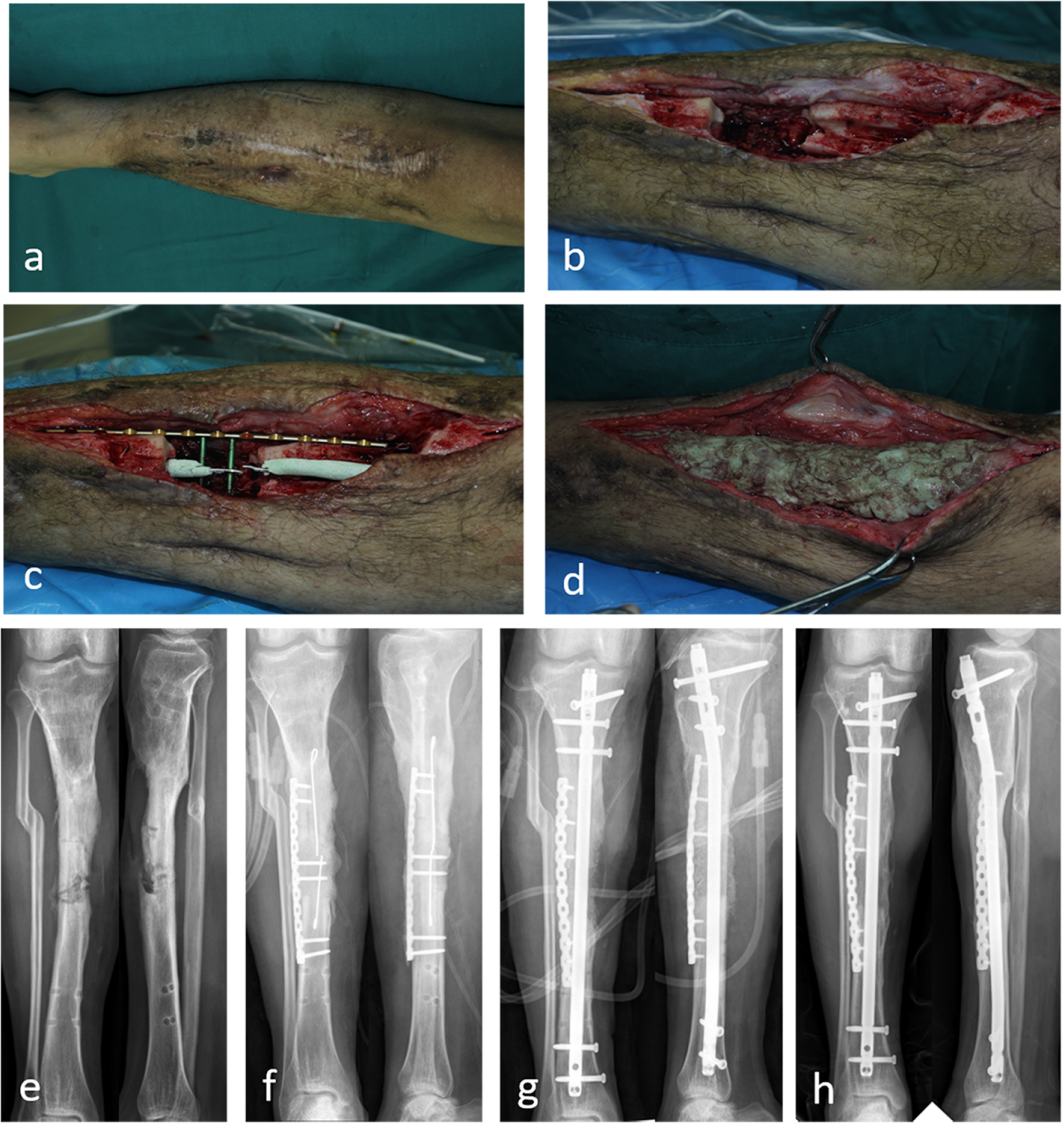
Twenty-three-year-old male. Bone infection after open reduction and internal fixation for 3 years. Deep tissue culture revealed *Staphylococcus aureus*. a Preoperative photo. b After debridement. c Reconstructive locked plate fixation. d Antibiotic bone cement filled with the defects and wrapped the fixation. e Preoperative X-rays. f X-rays after the first stage. g X-rays after the second stage. h Bone union after 36 months
